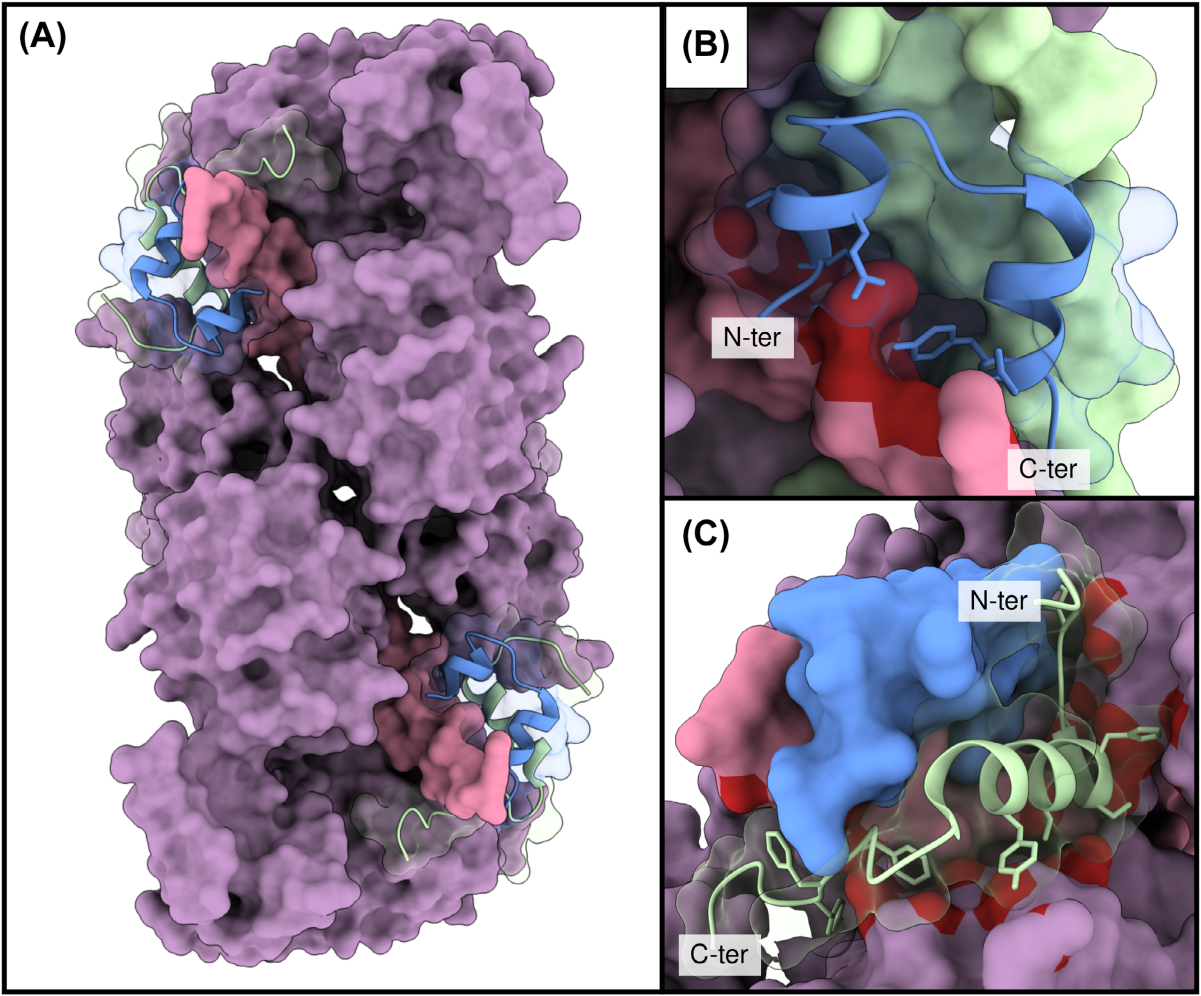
Structural analysis of insulin bound to a dimer of the α‐chain of the insulin receptor (IR). (A) Two insulin molecules are bound to the IR's α‐chain homodimer. The N terminus portion of the IR's alpha‐chain is colored violet, while the C terminus (α‐subunit C‐terminal helix, α‐CT) is colored pink. Insulin's A and B chains are colored blue and green, respectively. (B, C) Focus on the interaction surfaces. The red color represents the IR residues interacting with insulin. (B) The insulin A chain interacts most tightly with the α‐CT, especially through the peptide N‐ and C‐terminal regions. (C) The B chain establishes multiple distributed interactions, mainly with the N terminus portion of the receptor. Structure source: 6CE9 (PDB) [44].
